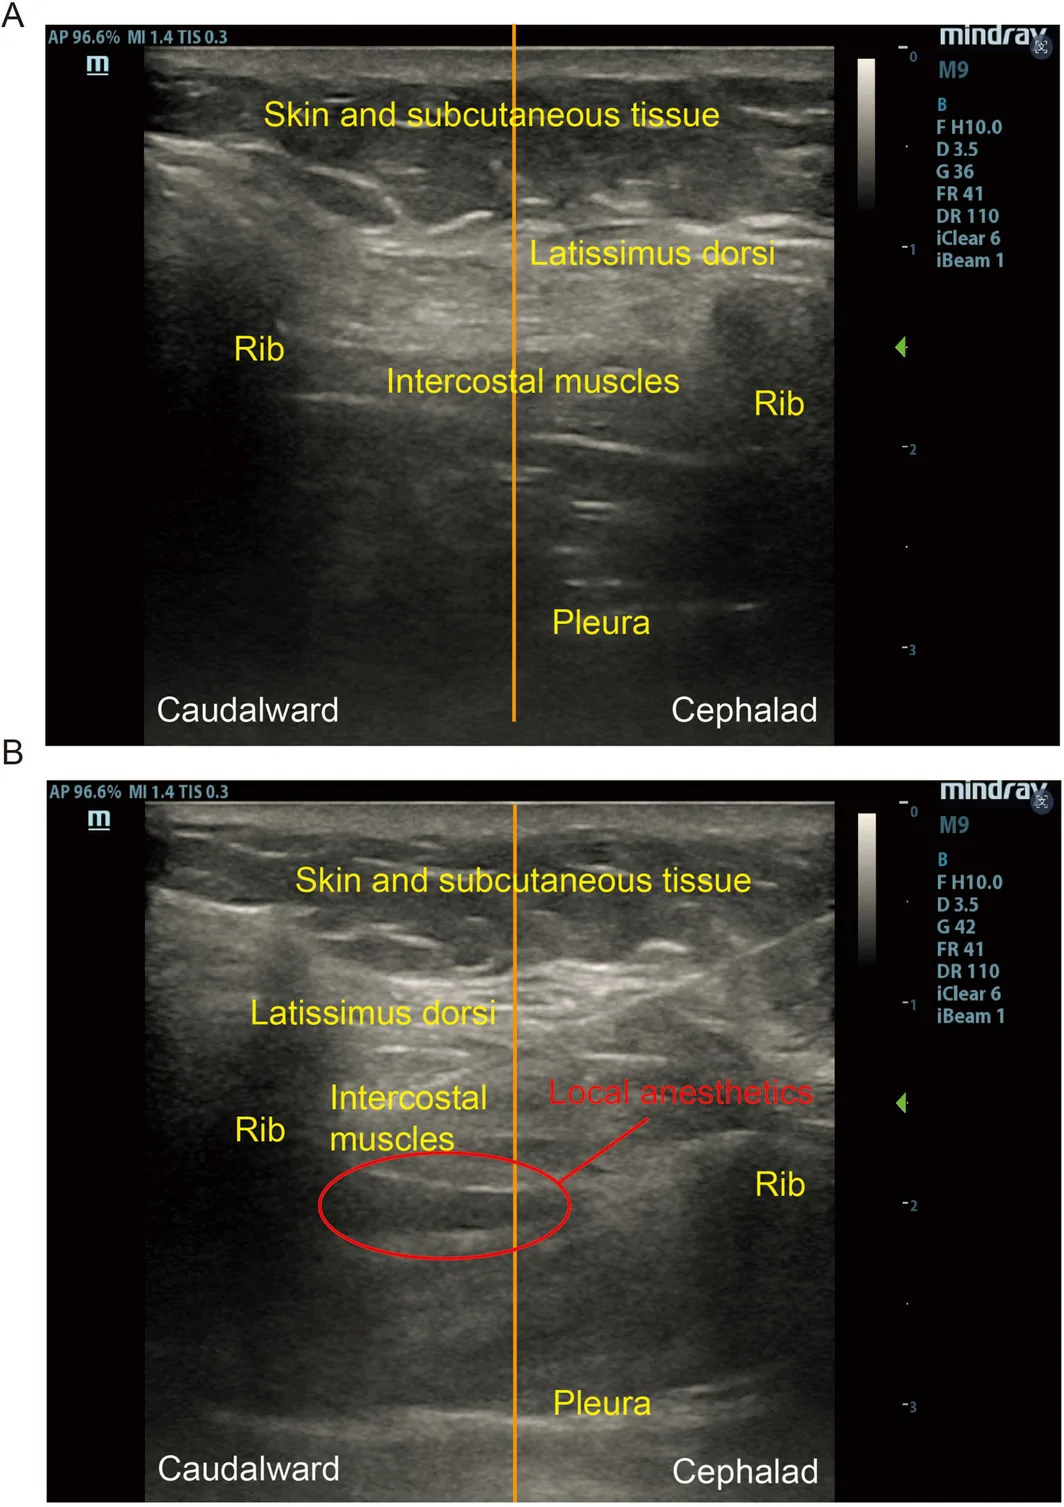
ICNB under ultrasound visualization before (A) and after (B) injection of local anesthetics.
